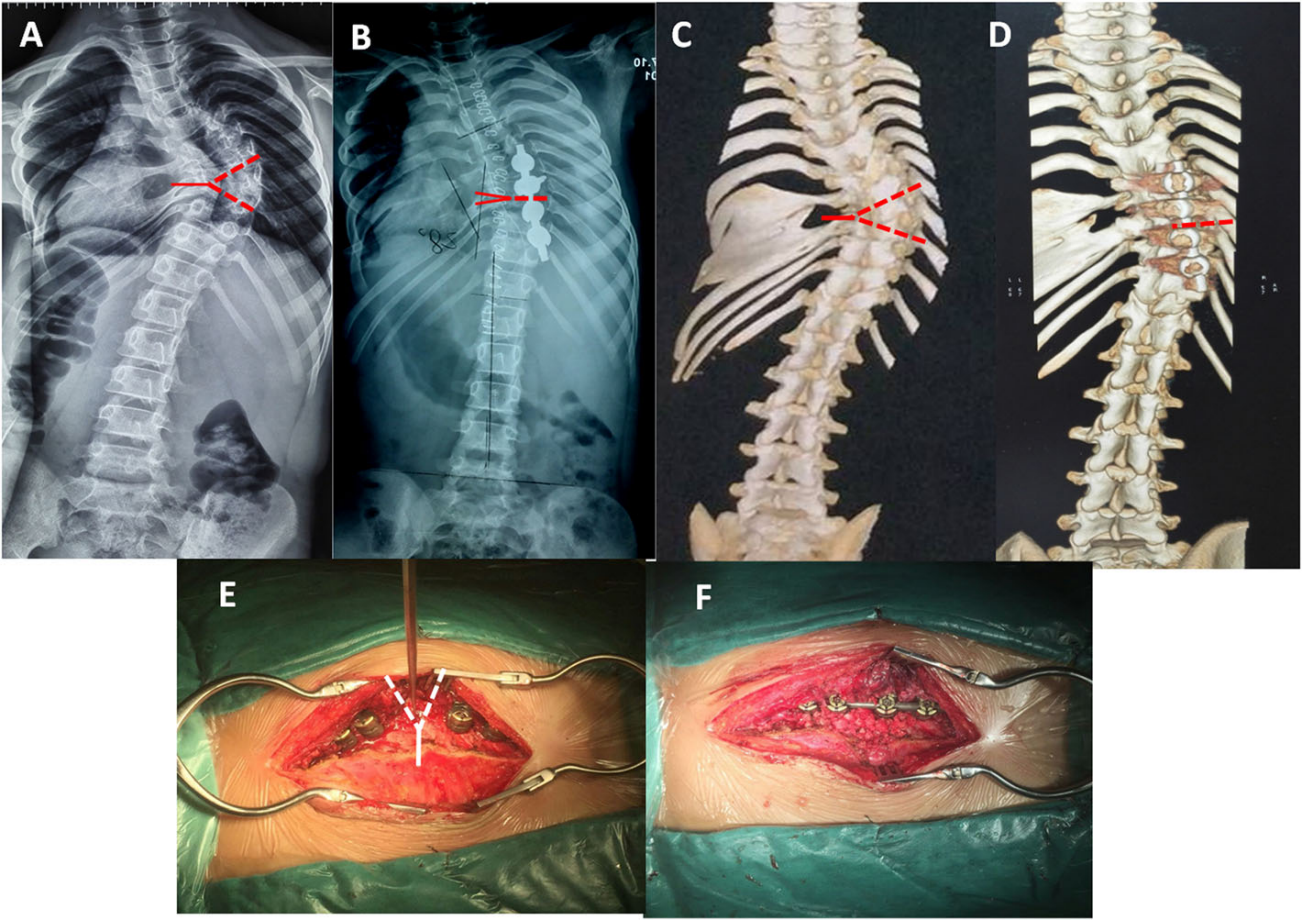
Pre- and Post operative photographs of a 10 year old patient who presented with complex congenital scoliosis; 3 dimensional CT showed failure of T5-9 anomaly vertebrae segmentation and massive fused rib; T8 Y-shaped osteotomy was performed with unilateral fusion (A,B,C,D). Interoperation image: convex side only to expose and unilateral pedicle screws were inserted. Gradual compression until the gap was closed after osteotomy. Bones graft for fusion of the posterior elements(E,F)
